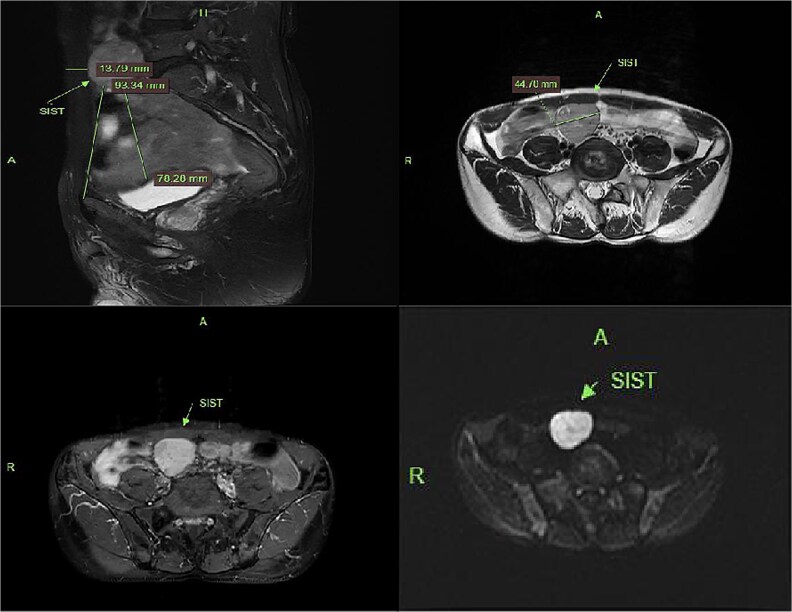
MRI demonstrates superior displacement of the tumor compared to CT, with isointense signal on T2-weighted fat-suppressed sequences, post-contrast enhancement, and hyperintensity on DWI at a b-value of 800 s/mm².
